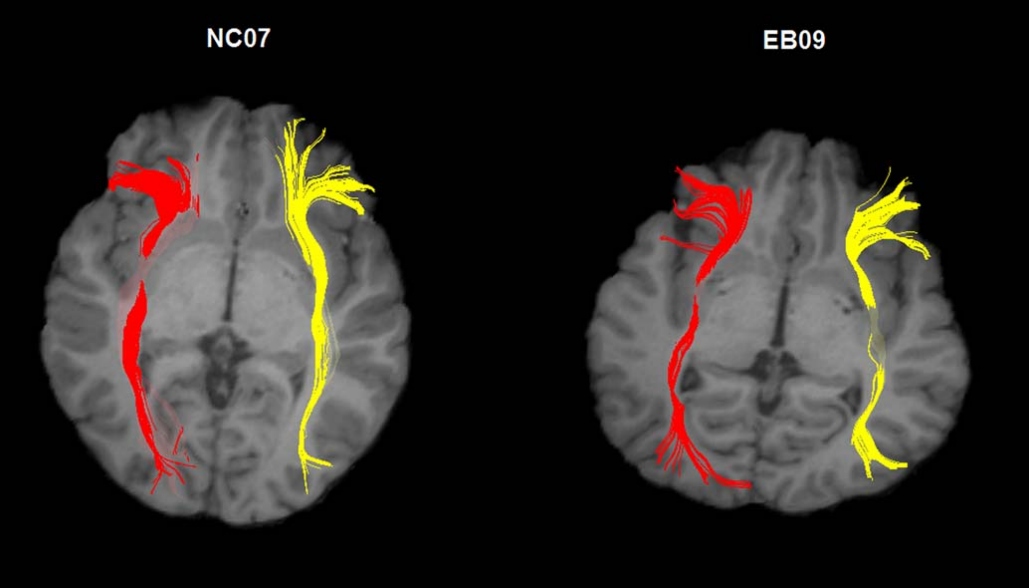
Representation of the reconstructed IFO fasciculi for a normal and blind subject. The tracked fibers are overlaied on the individual's anatomical image (T1-weighted). Left: normal subject NC07; right: blind subject EB09.
